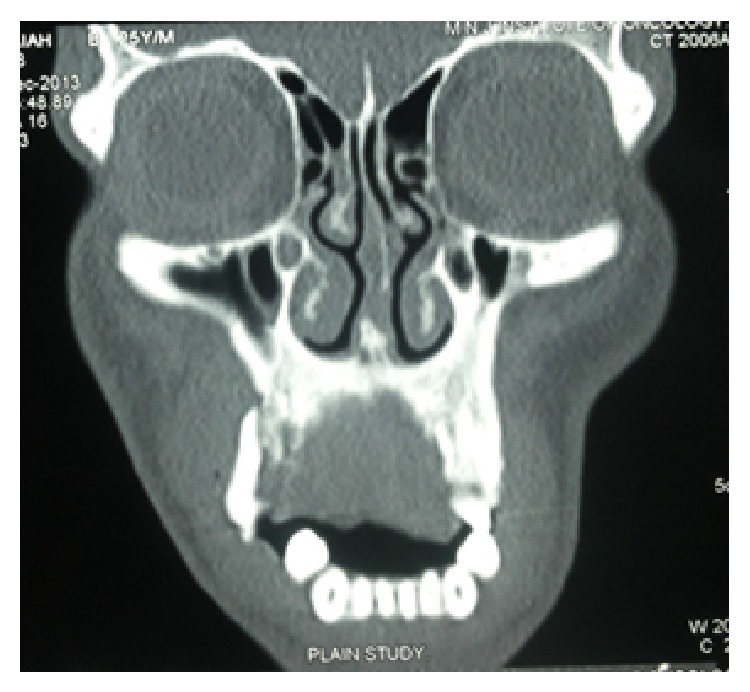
Coronal CT scan showing ill-defined enhancing soft tissue mass on palate with bone destruction.
